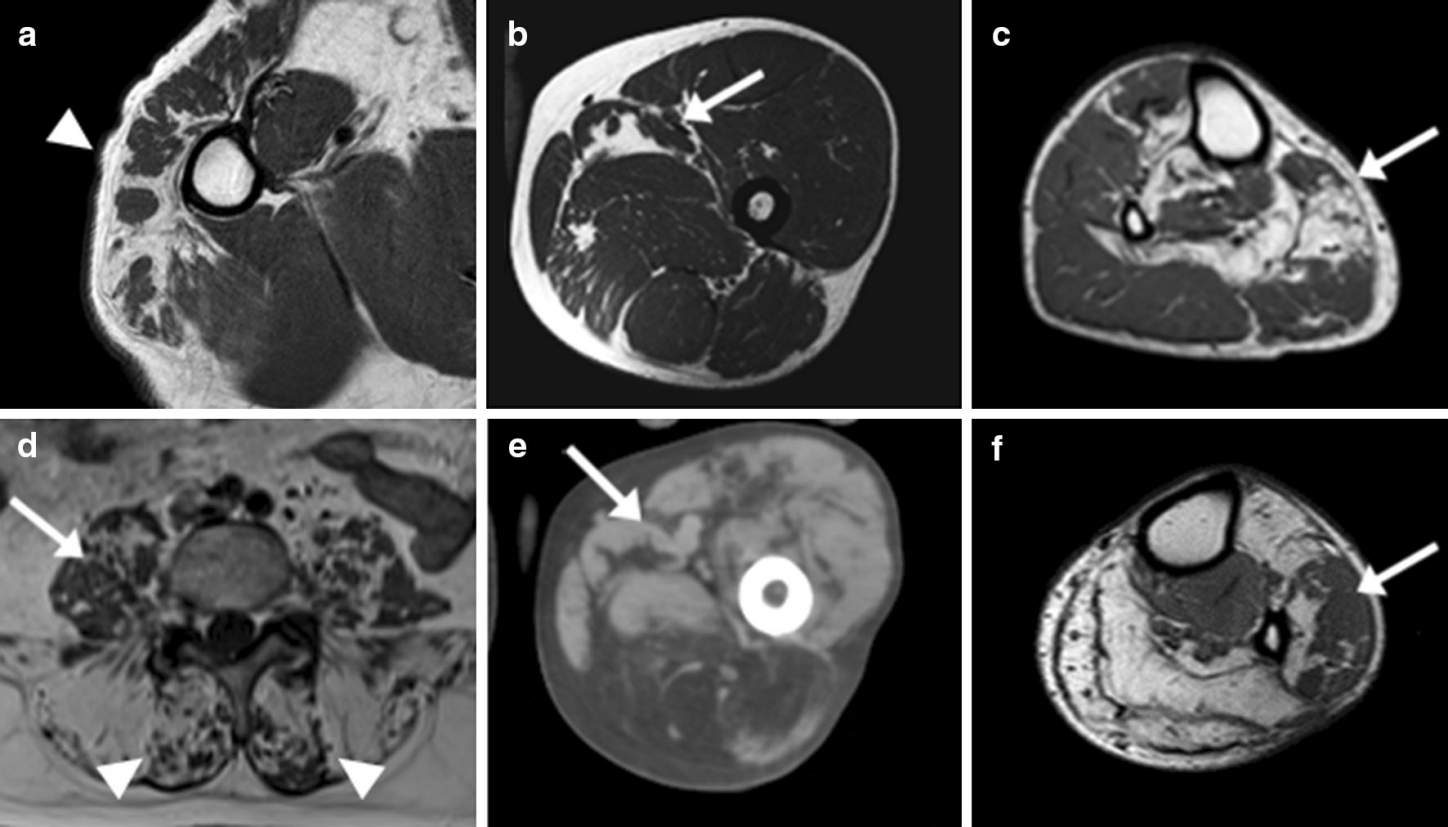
Patchy areas of fatty replacement in NLSD-M. T1-weighted images. a, b, f patient 2; c patient 5; d patient 4; e patient 7. Not homogenous muscle involvement in several muscles of different patients. Patchy areas of fatty replacement in bigger areas of not affected muscle (*arrows*) and muscle sparing areas in bigger fatty replaced areas (*arrowheads*)
